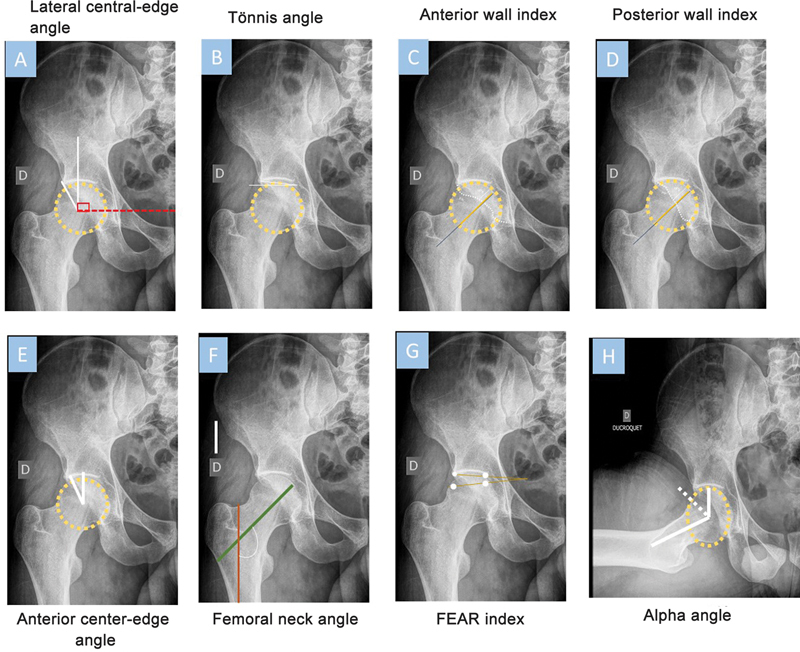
Radiographic measurements to identify borderline hip dysplasia and secondary factors, including hip coverage and instability.
